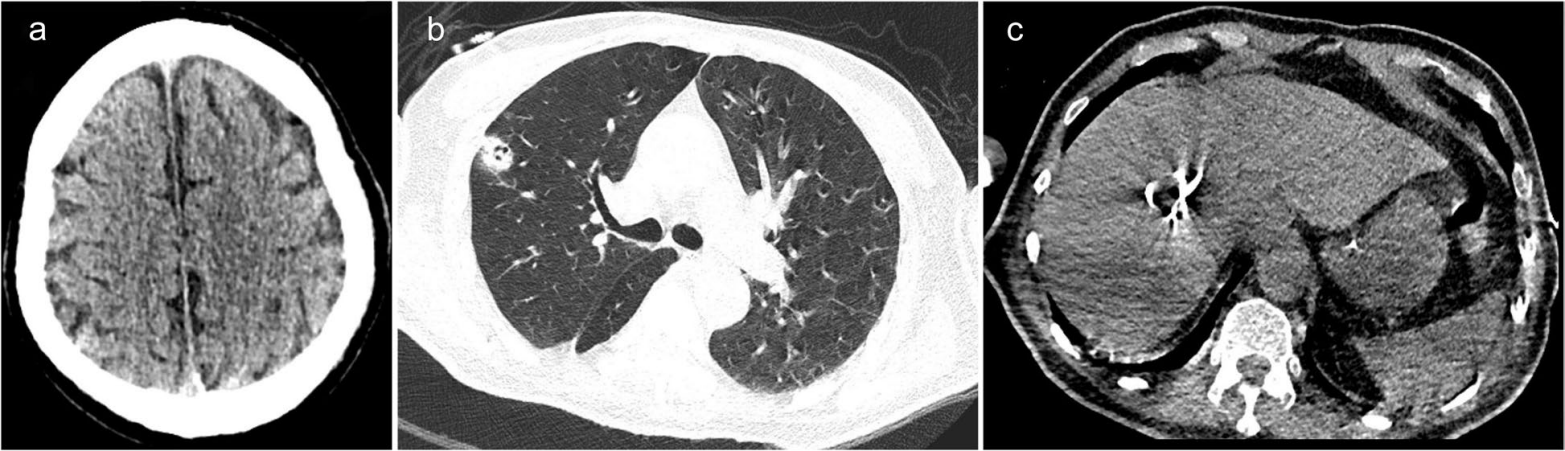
On day 7, the head CT scan showed hypodense lesions around bilateral frontal lobes, basal ganglia, and lateral ventricles, suggestive of lacunar cerebral infarctions (a). The chest CT scan showed bilateral pulmonary nodules with central cavitation. The largest nodule was located in the right upper lobe and measured approximately 23*17 mm (b). The abdominal CT scan revealed an abscess in the right lobe of the liver, with a maximum cross-section of 33.69*37.21 mm and catheter signs (c)
